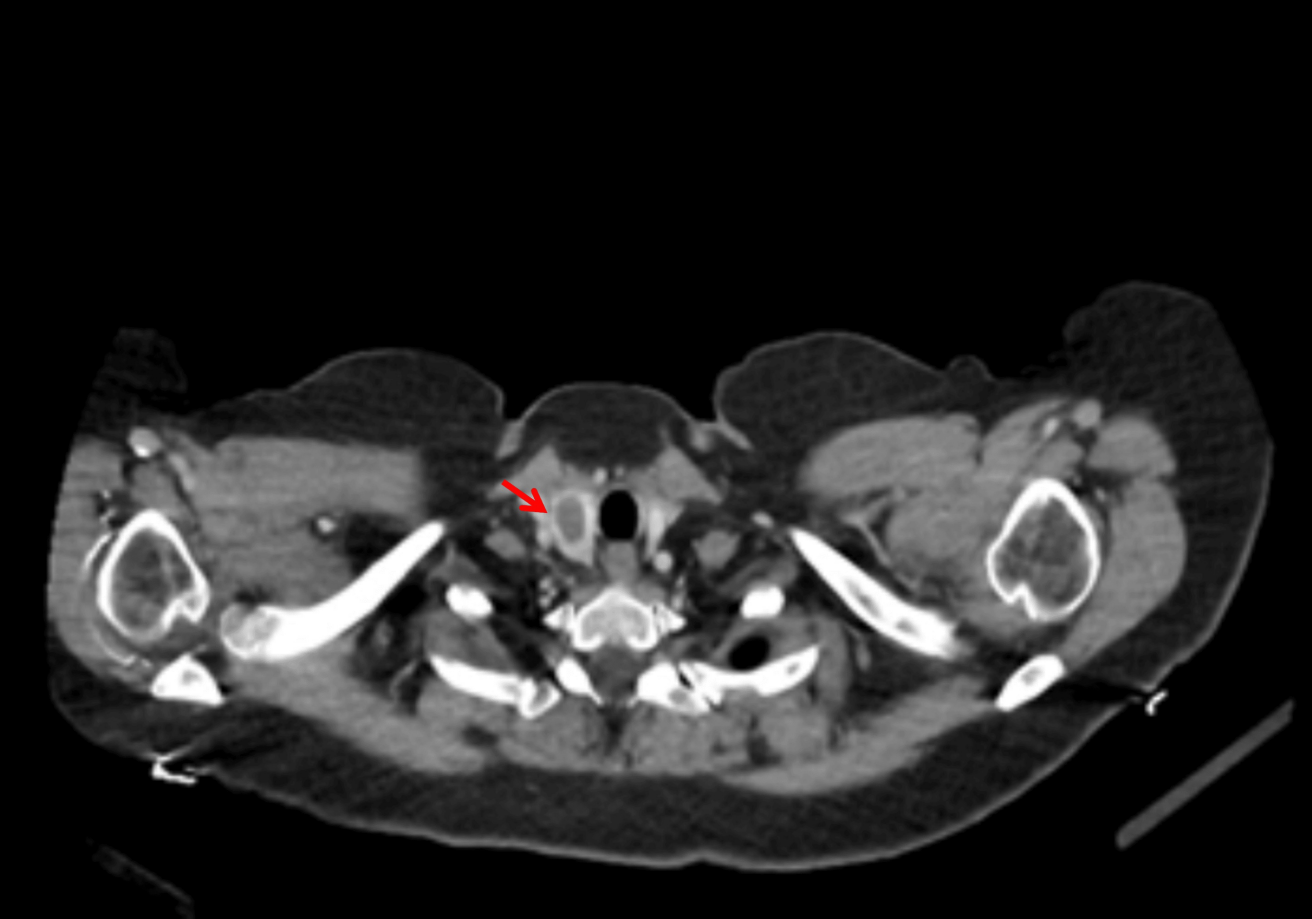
Computed tomography scan of the chest Red arrow indicates an enlarged right thyroid lobe
